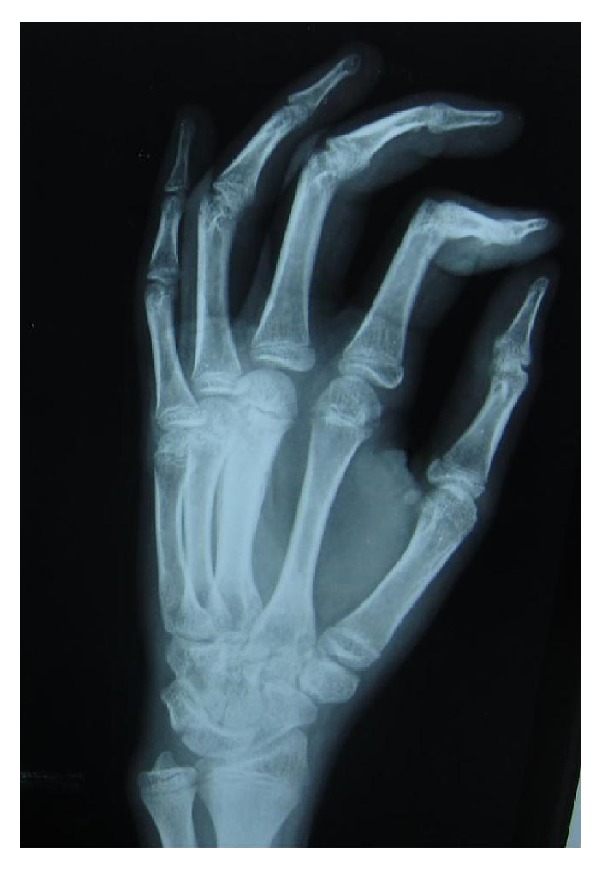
Radiograph of left hand (lateral view), showing cortical thickening and expansion of third metacarpal predominantly involving diaphysis with subperiosteal reaction.
